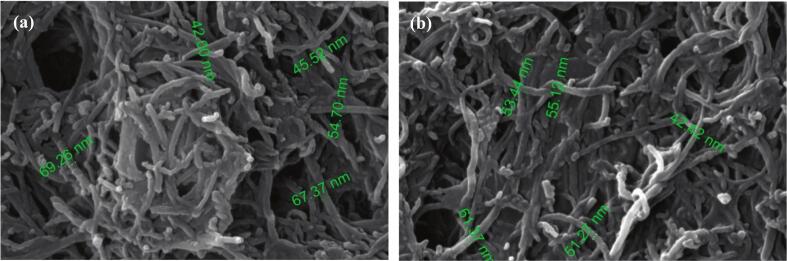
FE-SEM image of nanofluid after using in heat exchanger: (a) without ultrasonic waves, (b) with ultrasonic waves [86].
